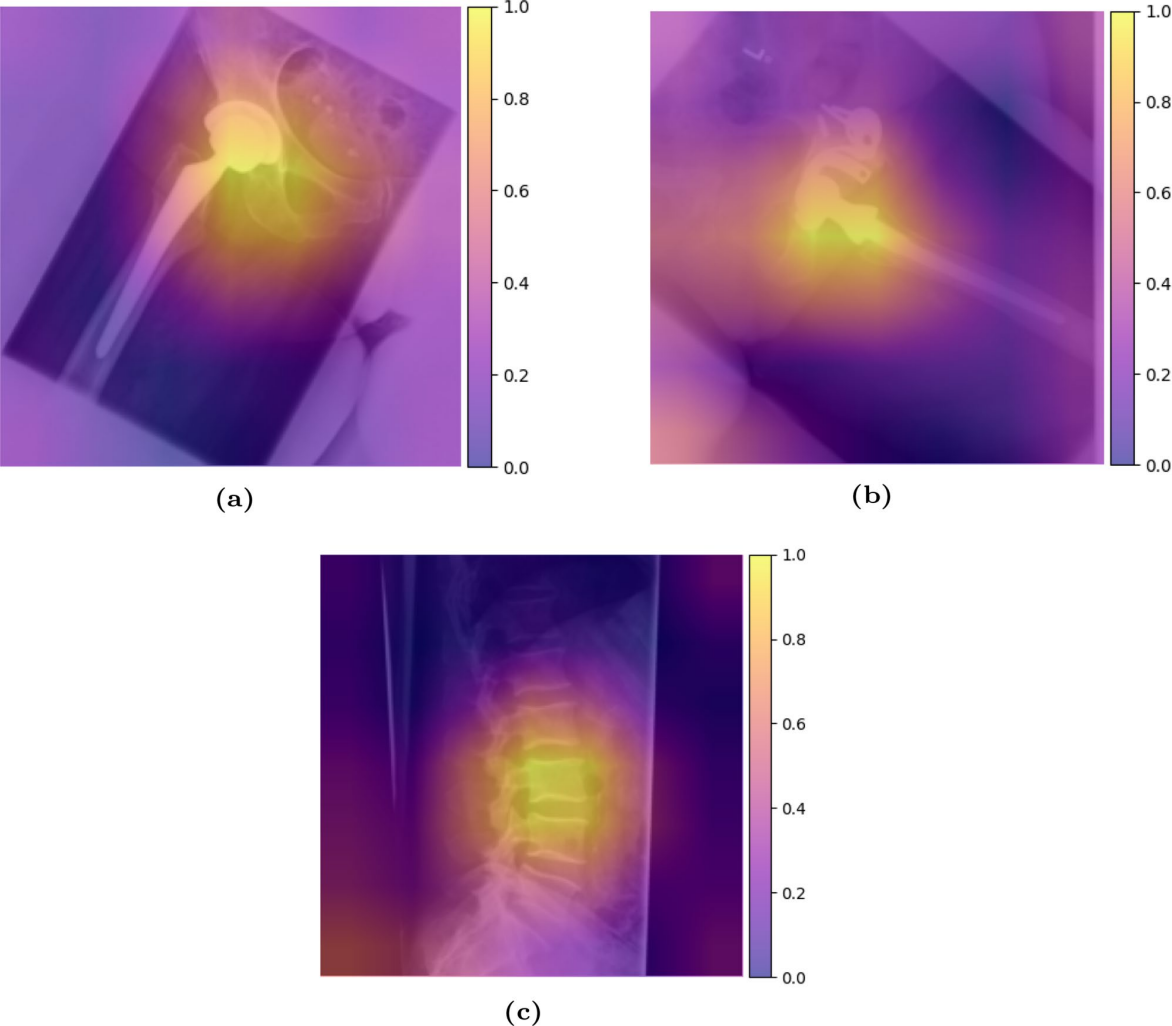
Cam of top 3 most confused test images. (a) True label = low extremity; prediction = hip. (b) True label = pelvis; prediction = hip. Black borders appearing on rescan X-rays may be caused by unexposed film edges, improper scanner alignment, or spacers used during the digitisation process. c) true label = thoracic spine; prediction = lumbar spine
